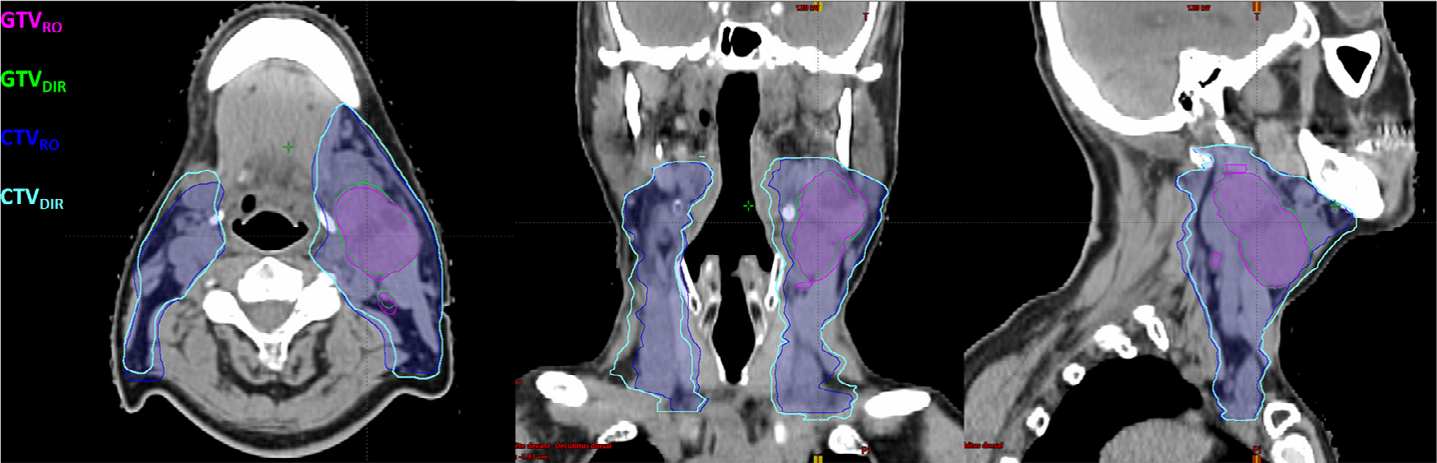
GTV and CTV manually edited by RO on replanning CT (GTV_RO_, CTV_RO_) and automatic propagation from initial CT to replanning CT using Smartfuse (GTV_DIR_, CTV_DIR_).
